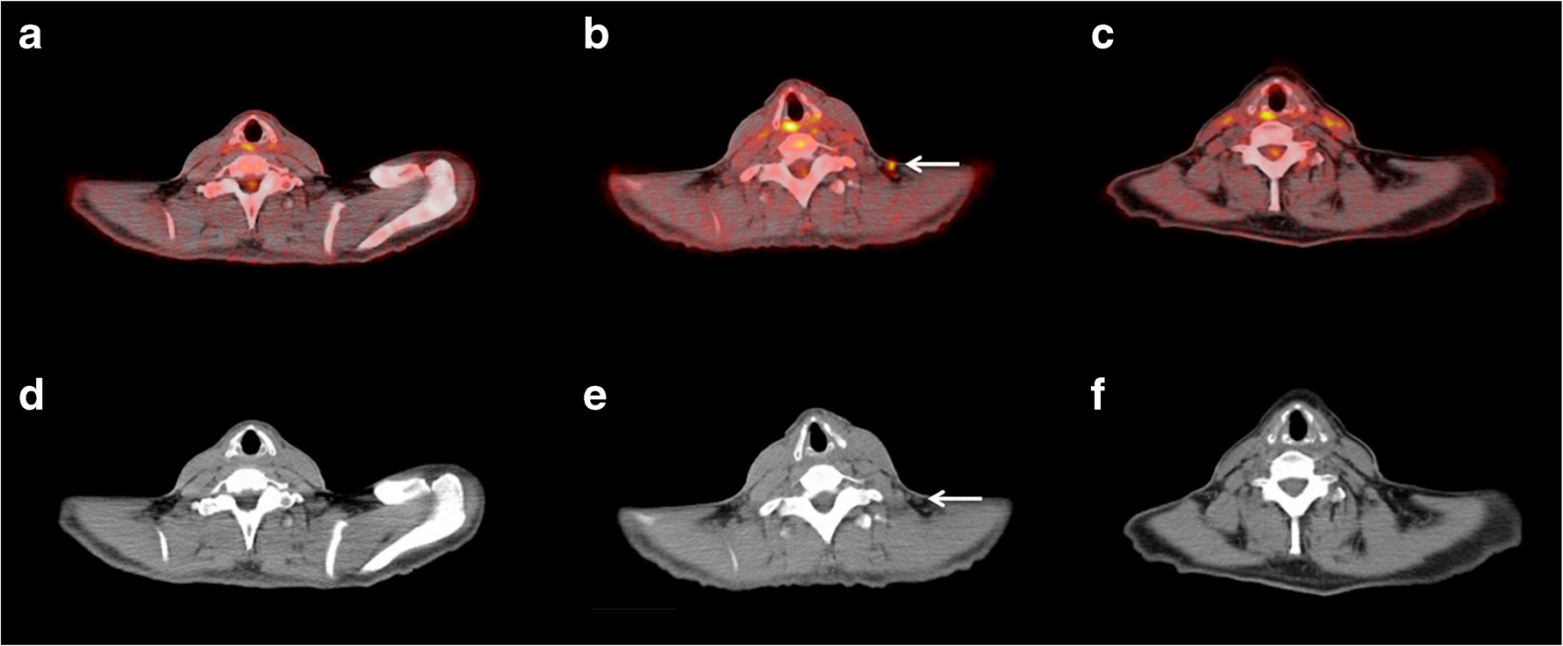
Transaxial PET/CT (upper row, A–C) and low-dose CT (lower row, D–F) images at the cervical level of a 48-year-old female patient with advanced melanoma. The PET/CT (A) and CT (D) images obtained before immunotherapy show no pathologic lesions. Interim PET/CT performed after two cycles of nivolumab/ipilimumab shows a new [^18^F]FDG-avid lymph node (white arrow; B, E), suspicious of metastatic involvement. According to the EORTC criteria, the patient showed progressive metabolic disease (PMD), while according to PERCIMT, he had stable metabolic disease (SMD). A third PET/CT obtained after administration of four cycles of nivolumab/ipilimumab shows remission of the lesion (C, F), suggesting pseudoprogression of the cervical finding on interim PET/CT. The patient had a PFS of 24.2 months and was still progression-free at last contact
